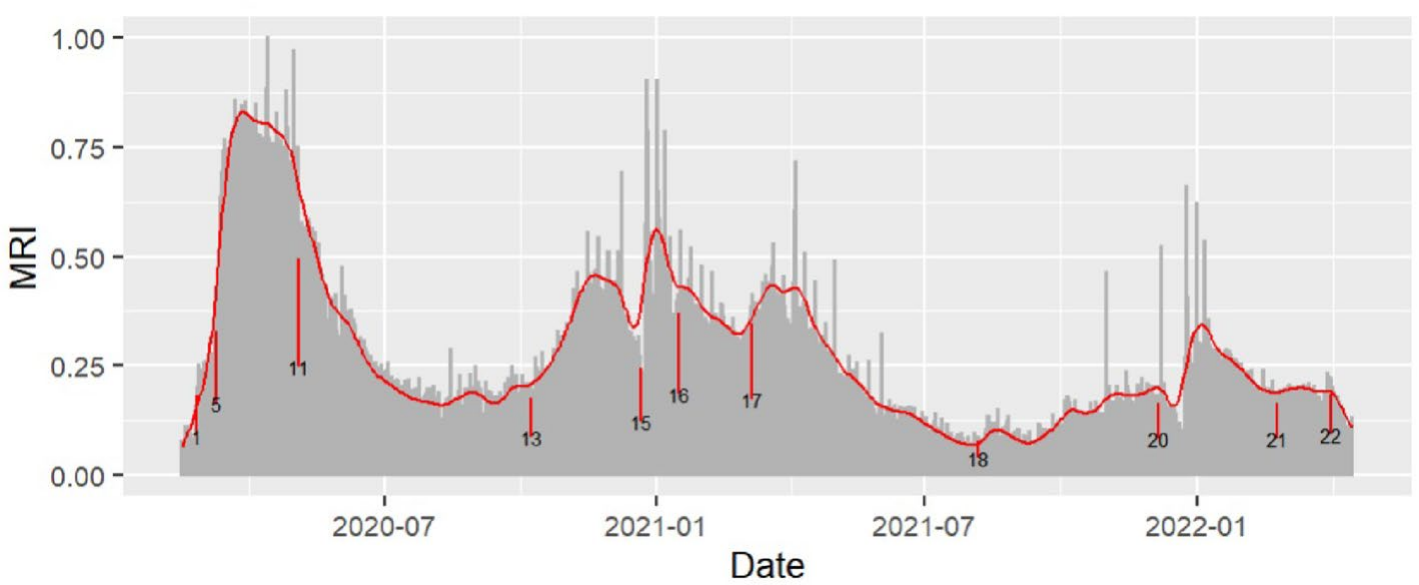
Time series of the MRI for Italy and major events from February 2020 to April 2022
